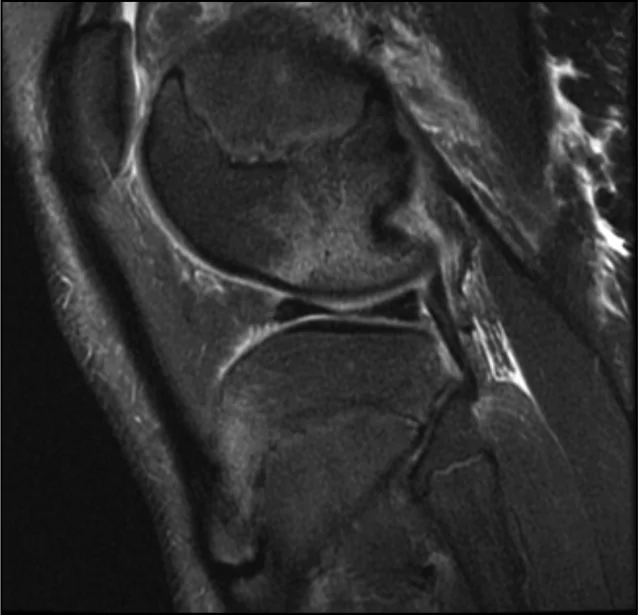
Sagittal MRI demonstrating PCL avulcion from the femoral attachement.
